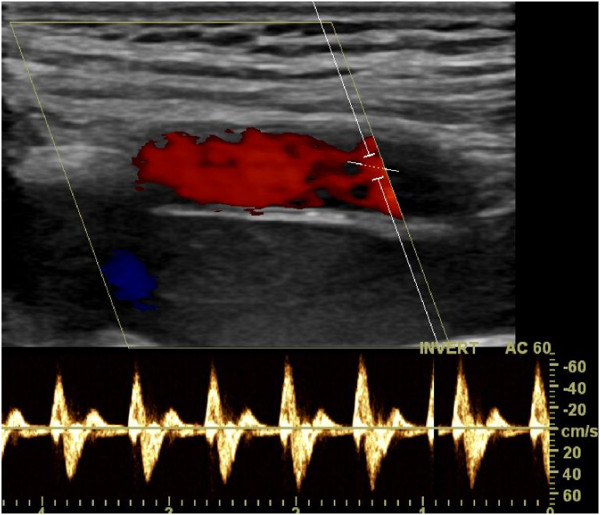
Postoperatively a Doppler ultrasound in neutral position as well as in 90 and 120 degrees of flexion is performed to rule out possible compression of femoral and epifascial vessels.
